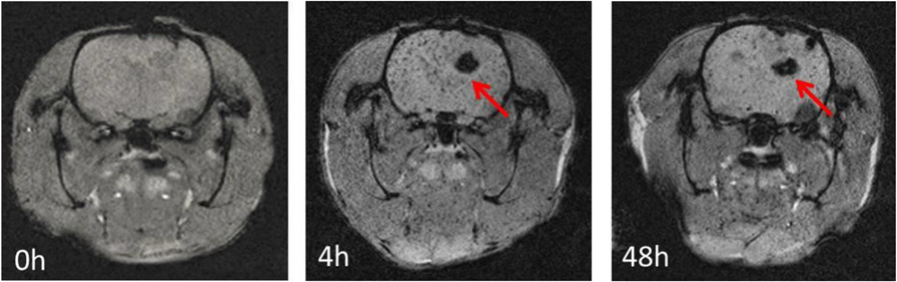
SWI MR images of gliomas before and post-injection of Lf-M-PAEEP-PLLA-NPs
